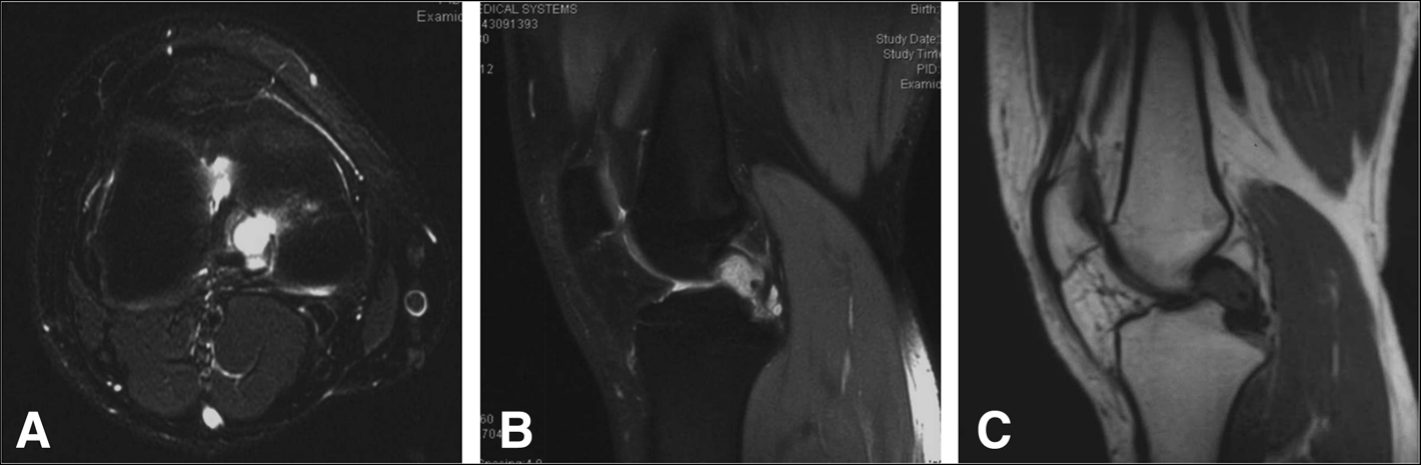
MRI of the intra-articular lesion in the right knee. a. T2-weighted axial MRI showed that the lesion was located between the condyles of femur with high signal intensity. b. T2-weighted sagittal MRI showed that the lesion was located in front of the posterior cruciate ligament with high signal intensity. c. T1-weighted sagittal MRI
